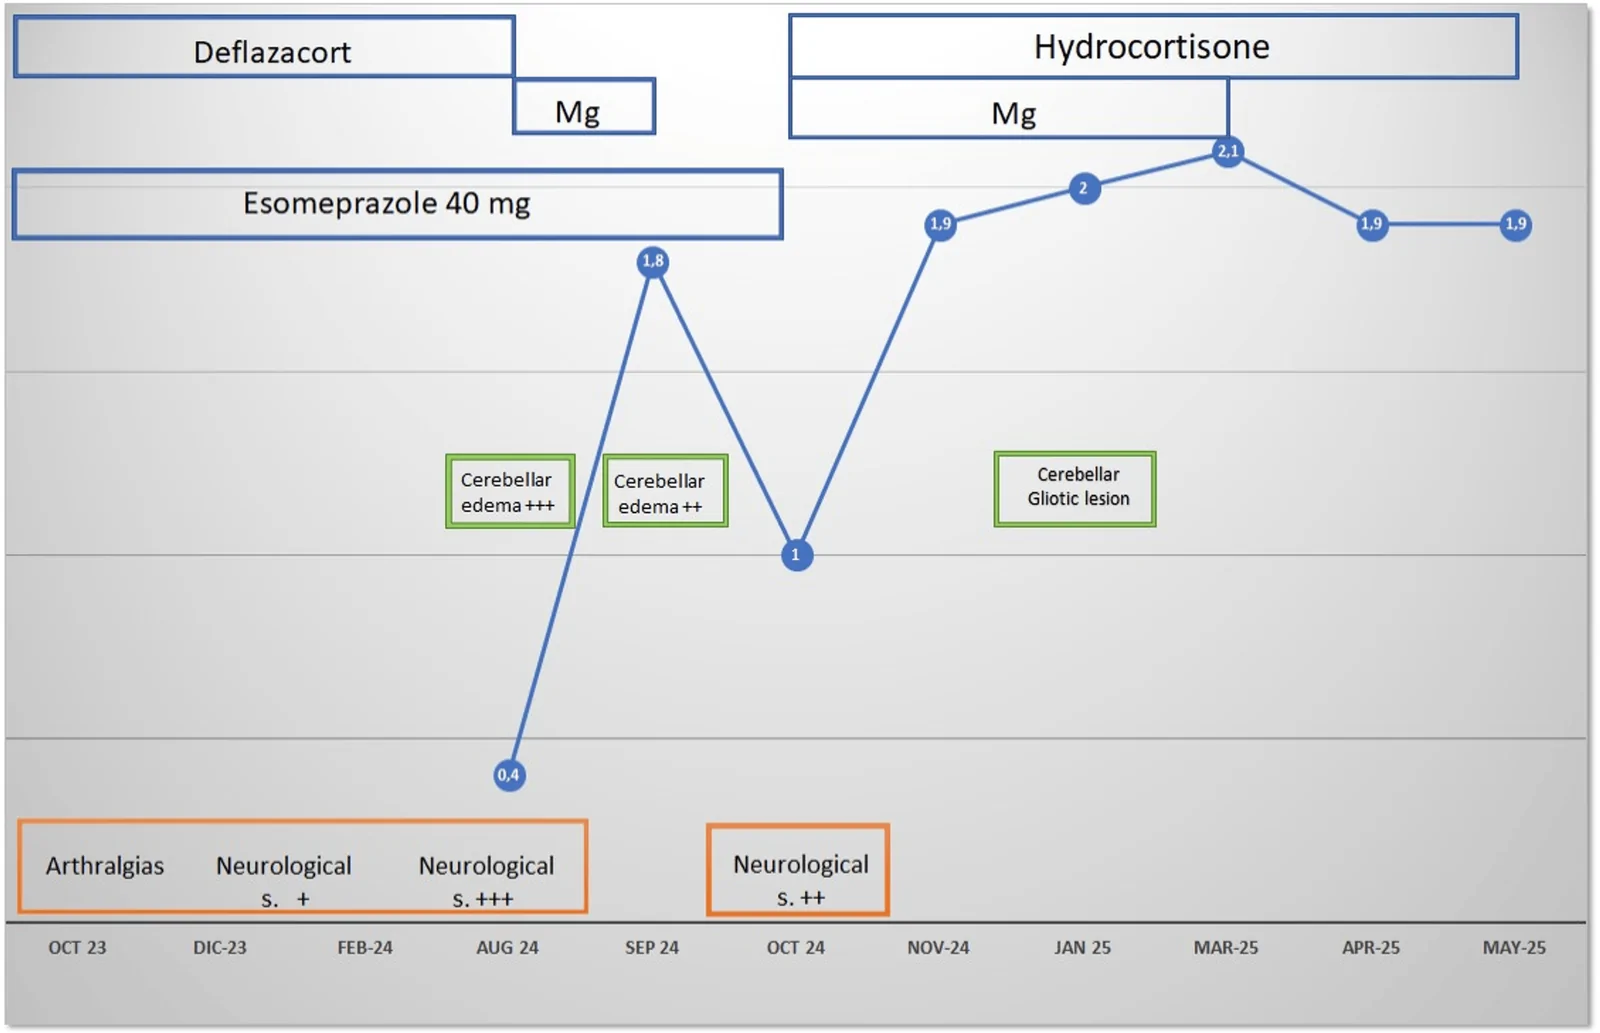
Time course of serum magnesium levels with therapies (blue boxes), MRI findings (green boxes), and clinical examinations (orange boxes).
